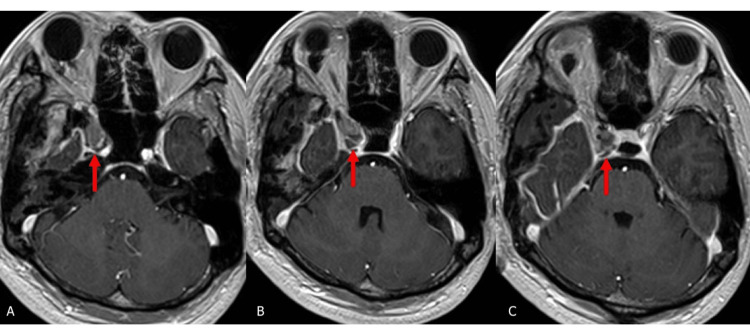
Postoperative MRI images. A-C: Gadolinium-diethylenetriaminepentaacetate (Gd-DTPA)-enhanced image showing postoperative status (red arrow).
